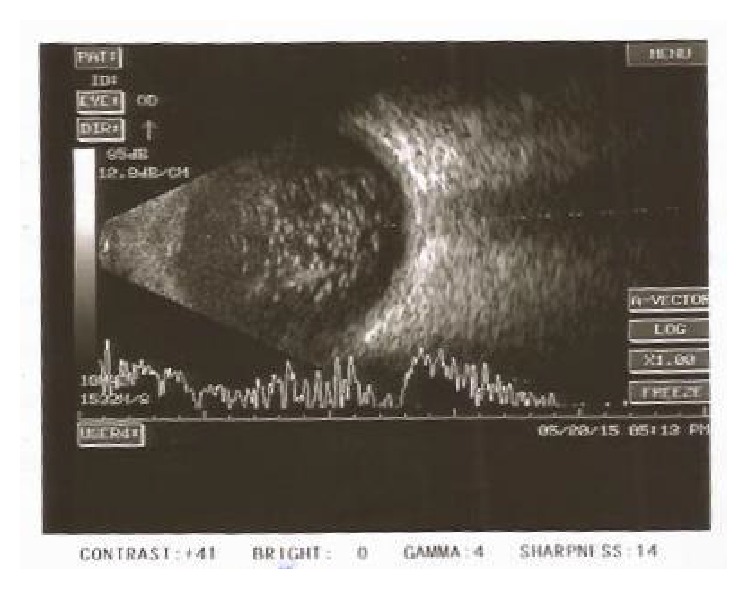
Left eye (Patient 2) B-scan ultrasonography shows resolution of choroidal detachment.
